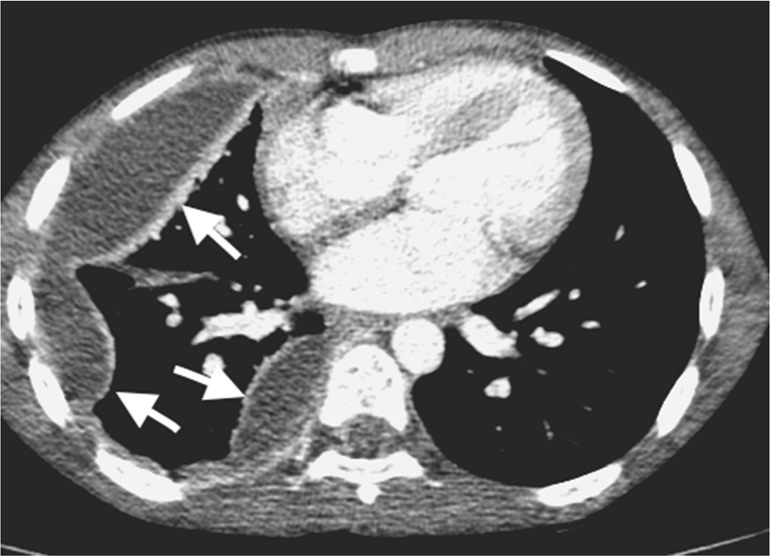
Contrast-enhanced axial CT of the chest in a 9-year-old girl demonstrates a thick enhancing rim associated with loculated pleural collections (*white arrows*), indicating an empyema, but there is no underlying parenchymal abnormality
